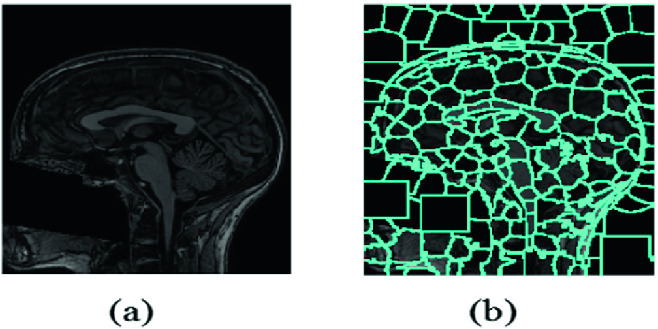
SLIC-based parcellation: (a) The input MRI. (b) Result of the SLIC method.
